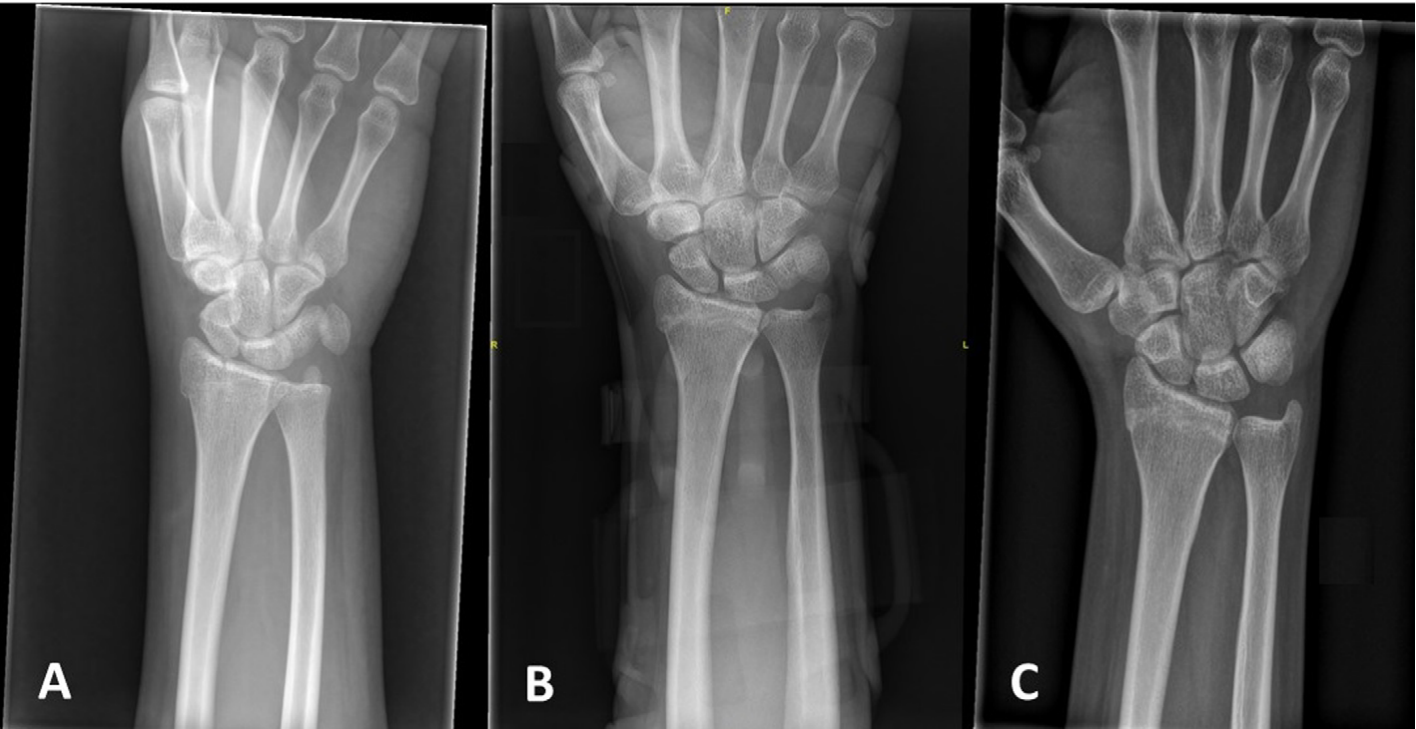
Radiographs demonstrating a radial styloid fracture of the right wrist at presentation to the emergency department. (A). Radiograph at the 6-week follow-up with the 3D-printed cast. Note that the fracture is not concealed, thus allowing for ideal follow-up. (B) Radiograph at 3 months showing union of the fracture with no loss of reduction. (C)
